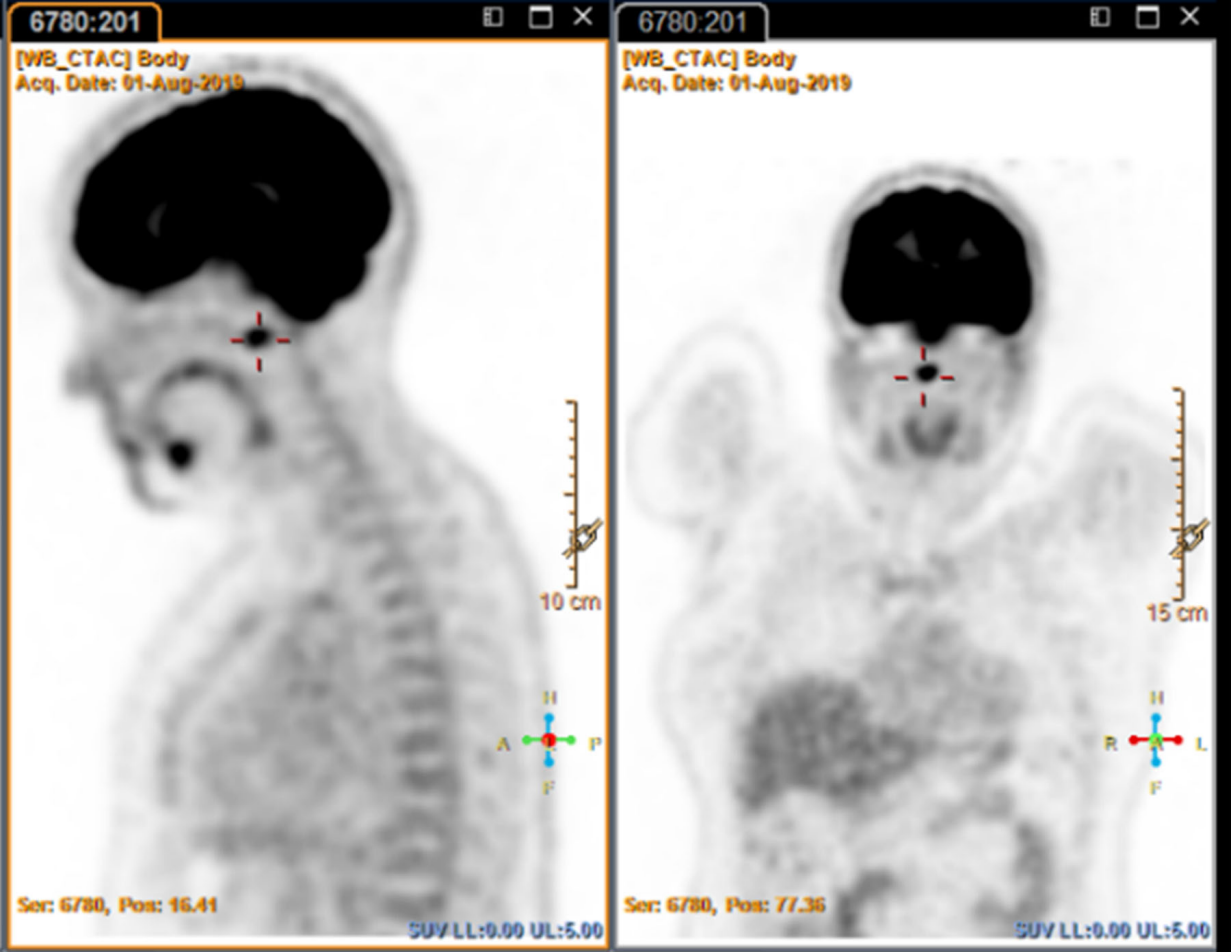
Case 1 PET scan. Moderate to intense FDG uptake in the upper cervical spine between the clivus and the anterior arch of C1.
